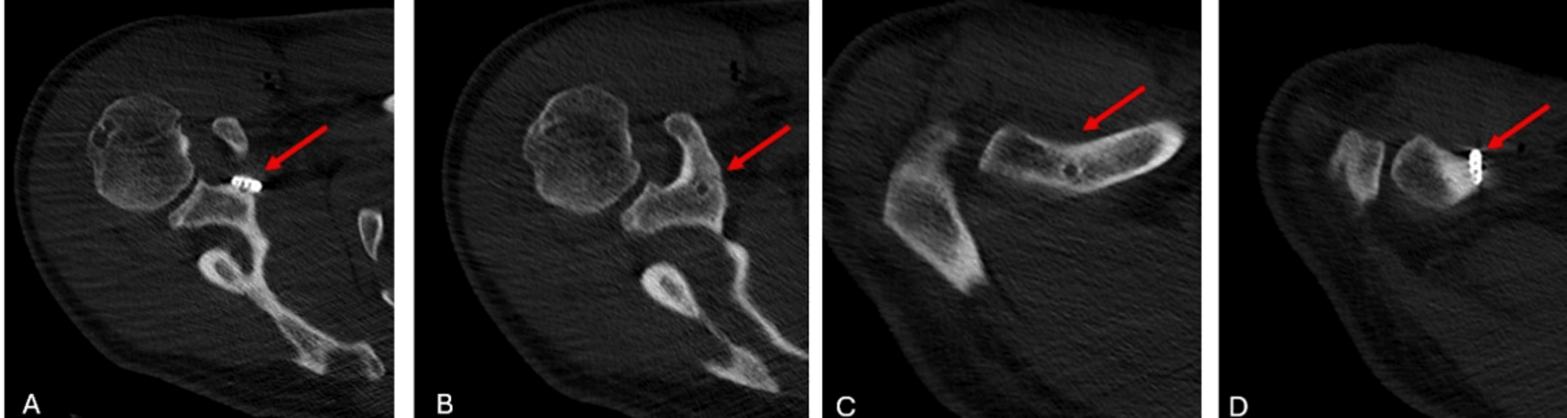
Postoperative axial CT images of the patient The images show the tunnel placement in the coracoid bone and clavicle, confirming proper implant positioning within the tunnel. The red arrows indicate the tunnel.
